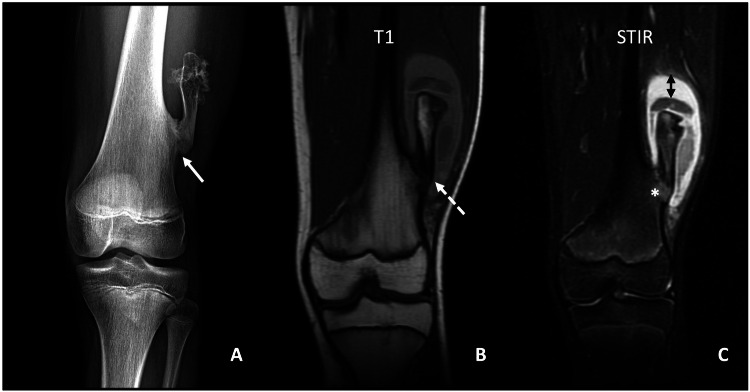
14-year-old male with a history of osteochondroma on the lateral aspect of the left distal femur, who consulted for pain after trauma. Conventional radiograph (A), coronal T1W MRI (B), and coronal STIR (C), showing the left distal femoral osteochondroma, with a radiolucent trace at the base (continuous arrow), compatible with fracture, which is also identified in the T1W sequence (dashed arrow). In the STIR sequence, there is an area of bone marrow oedema (*). Note the thick cartilage cap (< 2 cm thick) (double arrow). Resection of the osteochondroma was performed, with no signs of malignancy in the anatomopathological study
